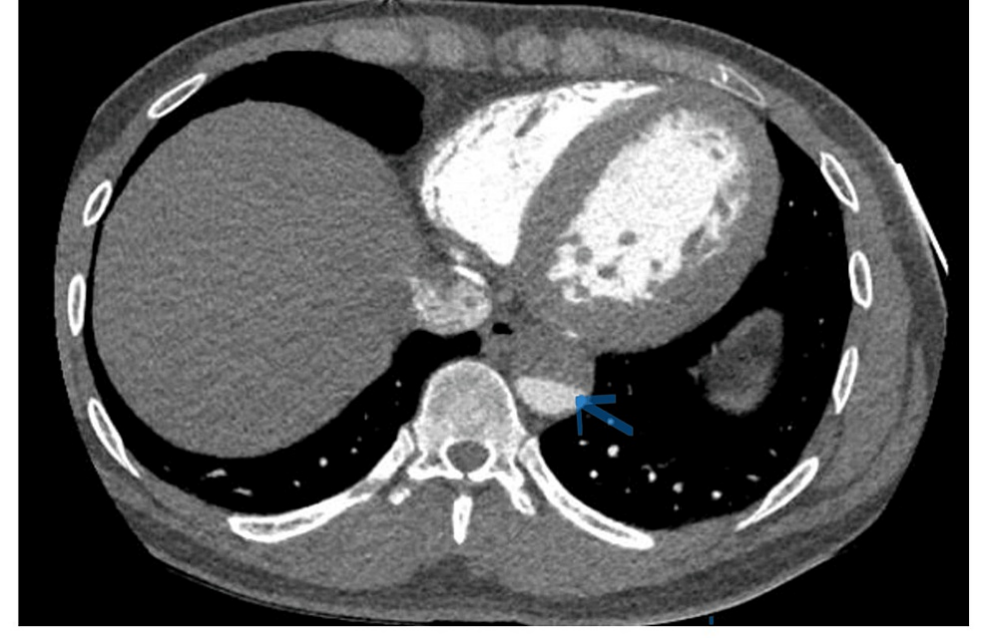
CT thoracic aorta on admission showing descending aortic dissection. True lumen (blue arrow).
